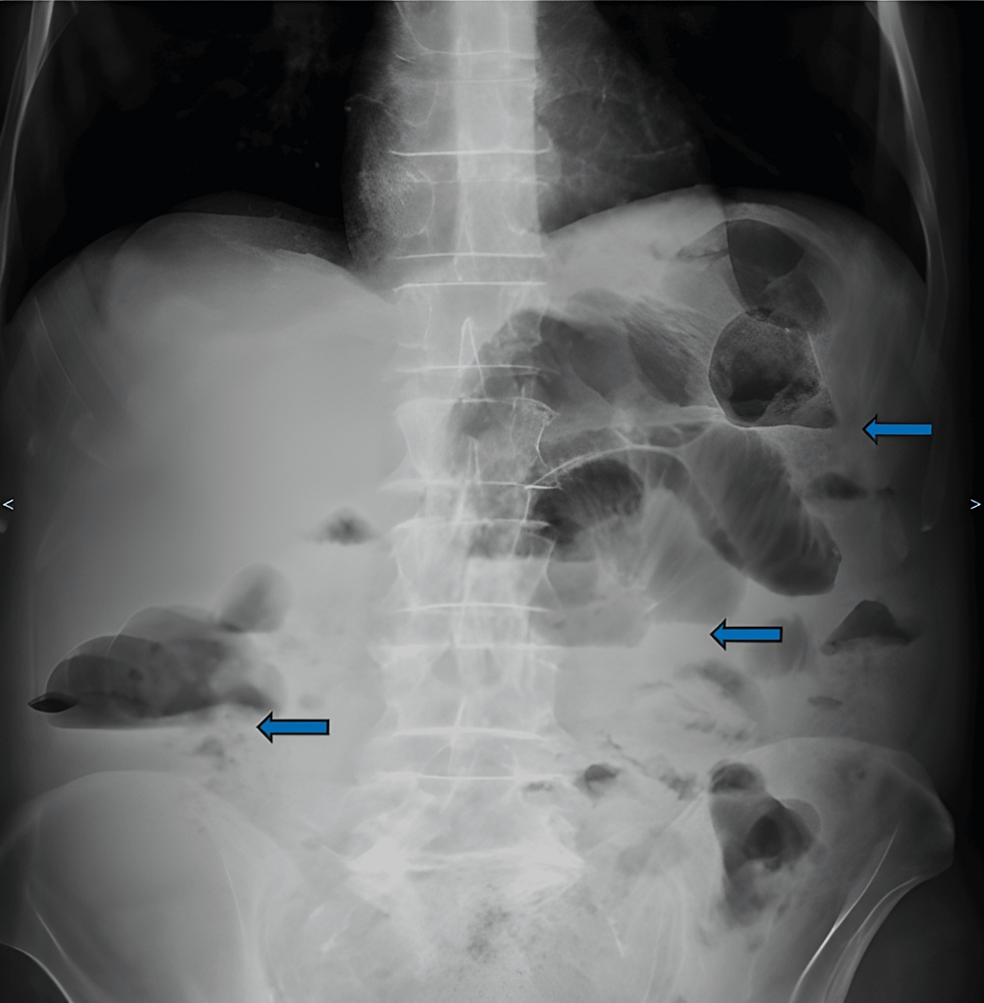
Abdominal X-ray Blue arrows: air-fluid levels
